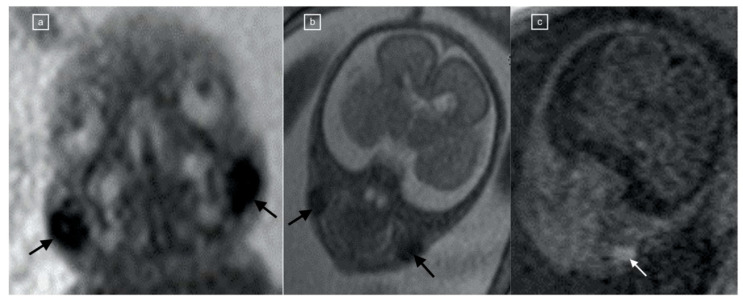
(a,b) Coronal TRUFI MRI in a 23-week fetus, one of dichorionic diamniotic twins, demonstrating bilateral symmetric, oval low-signal-intensity fat pads (black arrows) corresponding to (c) T1 VIBE hyperintensity (white arrow).
